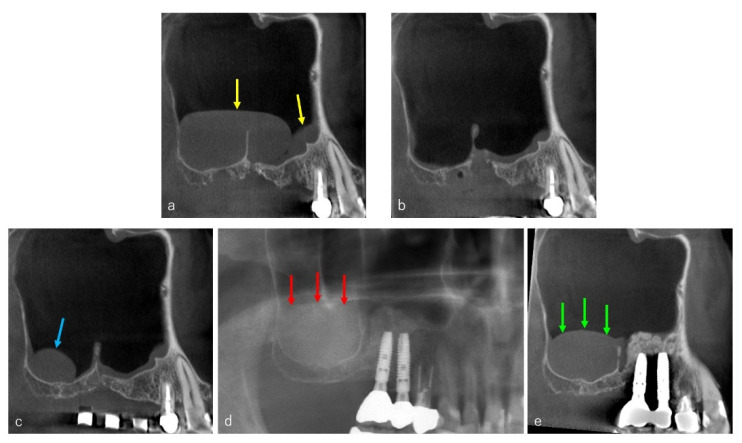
Case: 60 years old male. Two APs were observed, and the posterior AP existed across the septum (a), yellow arrows). They were removed, and the curettages of the extraction sockets were performed simultaneously (b). Three months after the removal, a relapse of AP had been occurred at the posterior area of septum ((c), blue arrow). Eight months after the removal, the sinus floor augmentation was performed only at the medial area of septum, and two implants were placed at the augmented area. Two point five years after the removal of APs, a panoramic radiograph ((d), red arrows) and a sagittal section CT image ((e), green arrow) showed the increased AP at the posterior area of septum.
